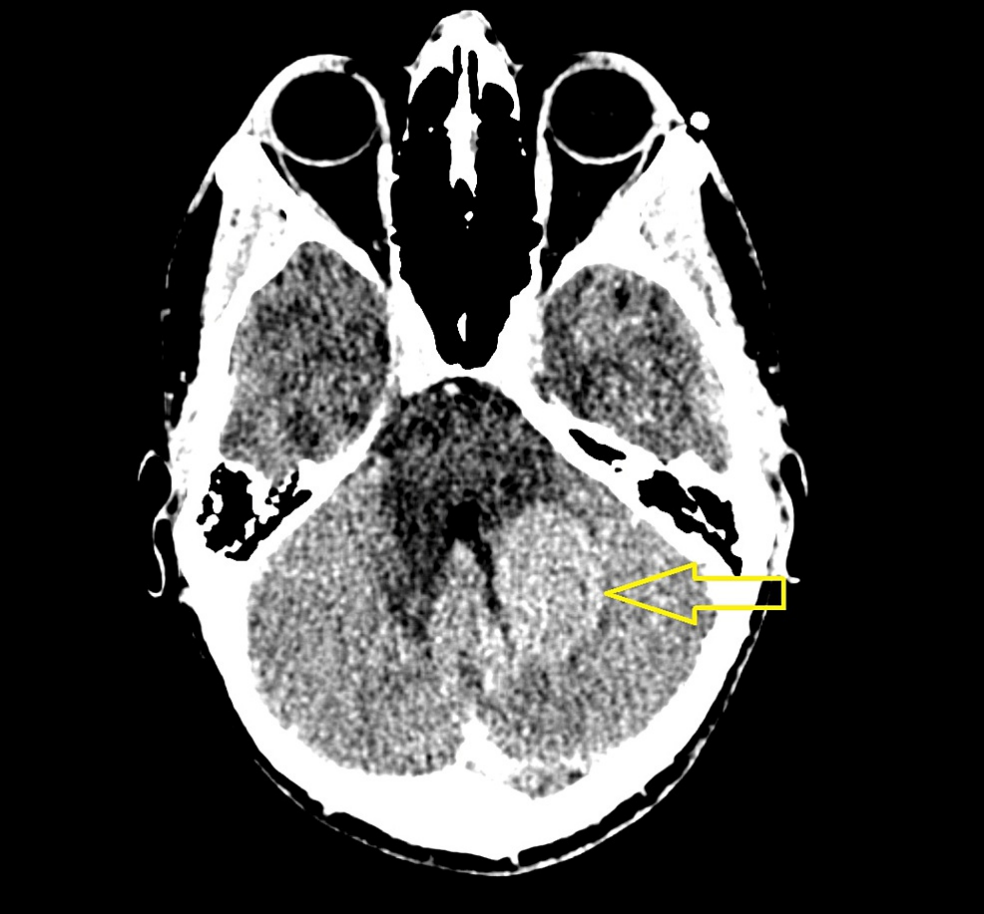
Contrast-enhanced axial CT scan demonstrating a mass in the left cerebellum. CT, computed tomography
